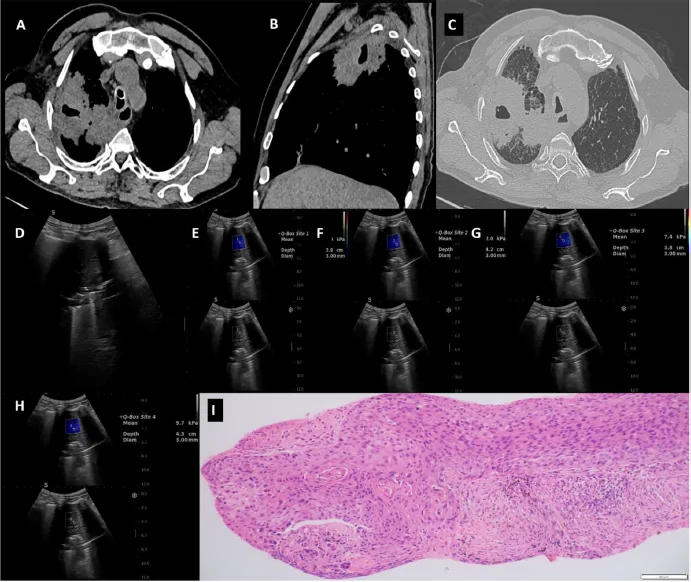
Mediastinal (A,B) and lung (C ) window sections of HRCT chest showing a spiculated cavitary lesion in the right lobe with elastography values of 10 kPa, 12 kPa, 7.4 kPa and 9.7 kPa. The mean came out to be 9.7 kPa. A biopsy revealed an invasive tumor arranged in nests, trabeculae and cords showing squamoid differentiation in the form of intercellular bridging and keratin pearl formation. Adjacent stroma shows a mild mixed inflammatory infiltrate. Features are of non-small cell carcinoma favoring squamous cell carcinoma – keratinizing type.
